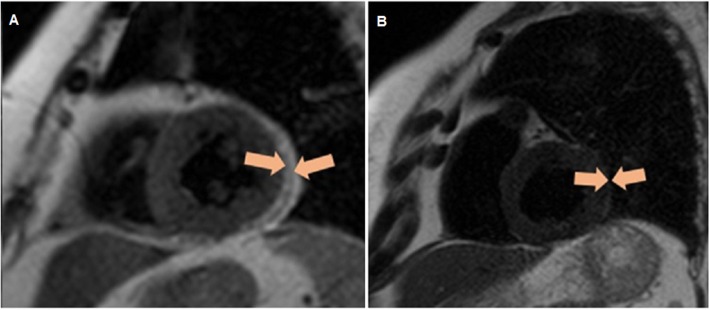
Quantitative assessment of the thickness of epicardial adipose tissue along the anterior wall of the right ventricle, as well as the thickness of the epicardial adipose tissue (orange contour) along the posterior wall of the left ventricle via magnetic resonance imaging. (A) Patient without obesity, (B) patient with obesity.
